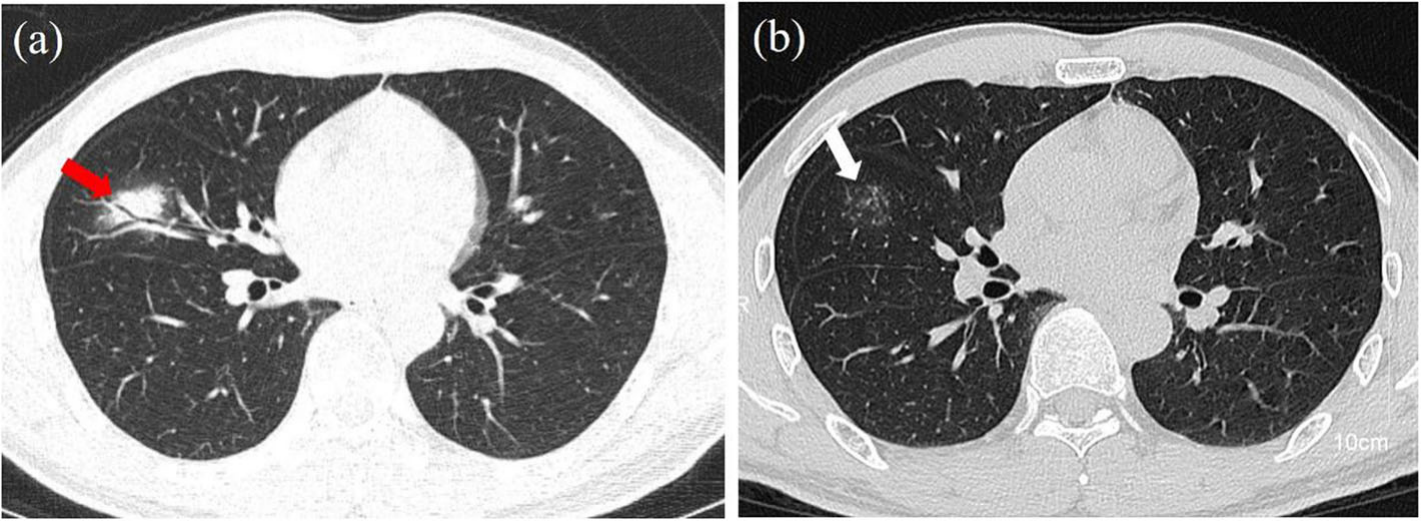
A male patient infected with SARS-CoV-2. a The first CT was performed three days after the onset of symptoms. The chest CT image shows single consolidation in the right middle lobe and air bronchogram (red arrows). b The second CT was performed 13 days after the treatment. The chest CT image shows a tree-in-bud pattern (white arrows)
